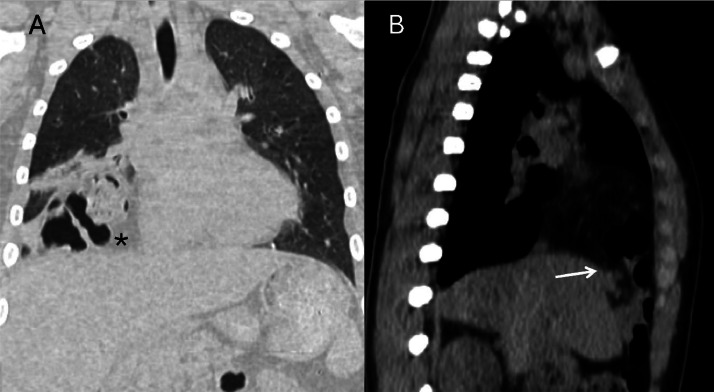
Computed tomographic scan (A: coronal plane; B: sagittal plane) showing bowel loops and omentum (asterisk) herniating through the right side of the diaphragm. In (B) the diaphragmatic defect is clearly seen, allowing to assess its extension (white arrow).
